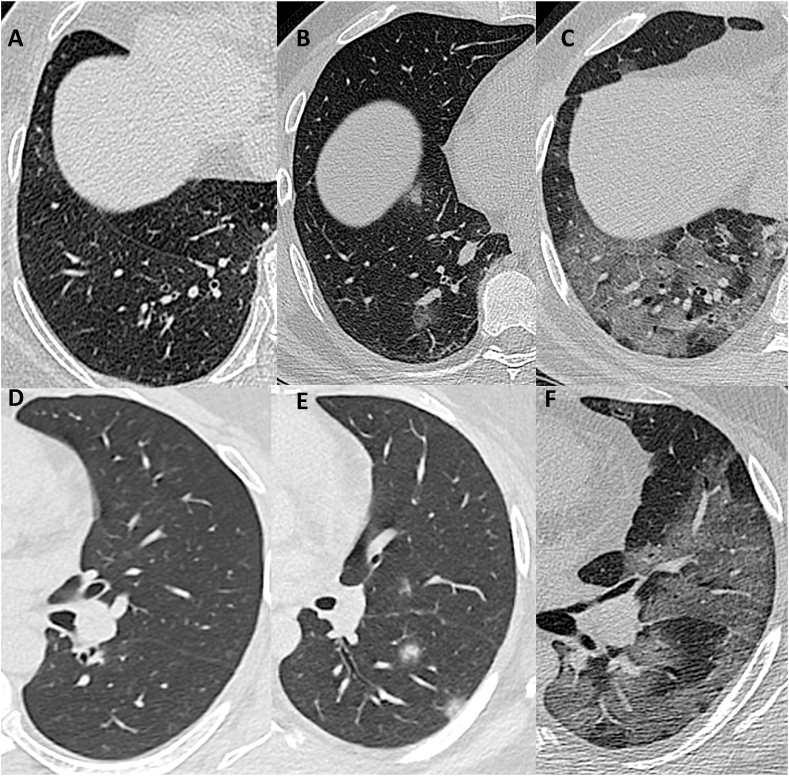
Representative axial non-contrast chest CT images from different patients to show the calculation of the CT-SS in the right posterobasal segment (S10) (A–C) and left superior segment (S6) (D–F). In images A and D, the absence of ground glass opacities or consolidation correspond to 0 points. In images B and E, less than 50% compromised segment corresponds to 1 point. In images C and F, diffuse ground glass opacities in more than 50% of the studied segments correspond to 2 points.
